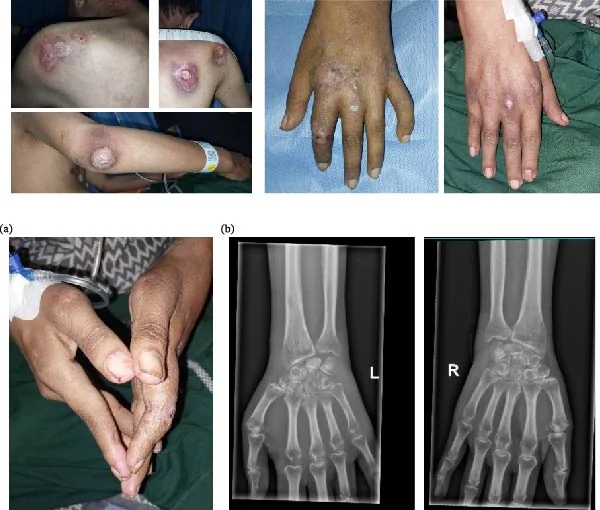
Multiple ulcers shown on right scapula, left and right shoulders and elbow and metacarpophalangeal joints of the fingers. (a) Hand deformity showing flexion at the PIP joint and hyperextension at the DIP joint of the 2^nd^ left hand digit. (b) Radiographic image showing juxta‐articular wrist osteopenia.
